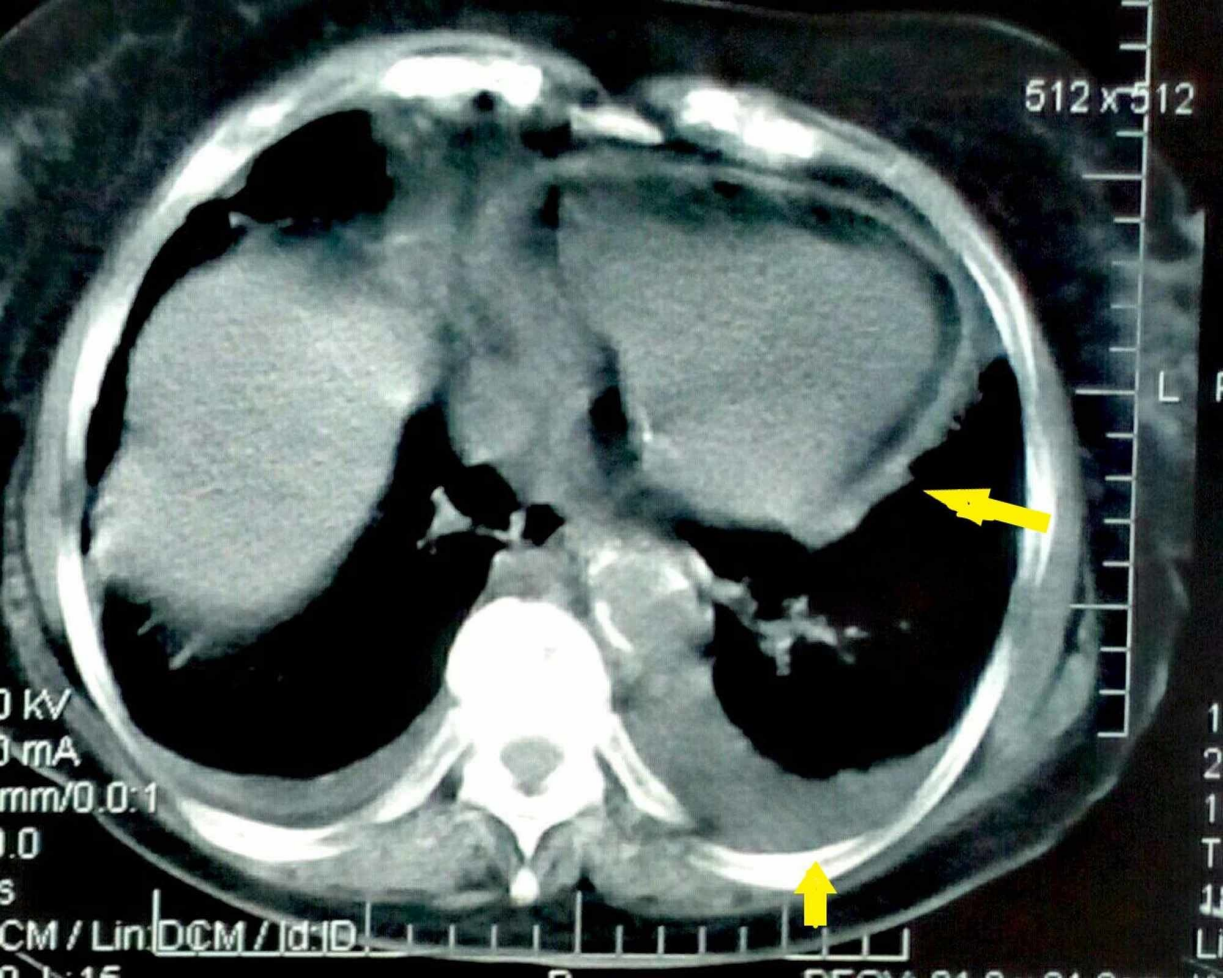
CT of the chest on admission showing a large left pleural effusion, small right pleural effusion, and small pericardial effusion
